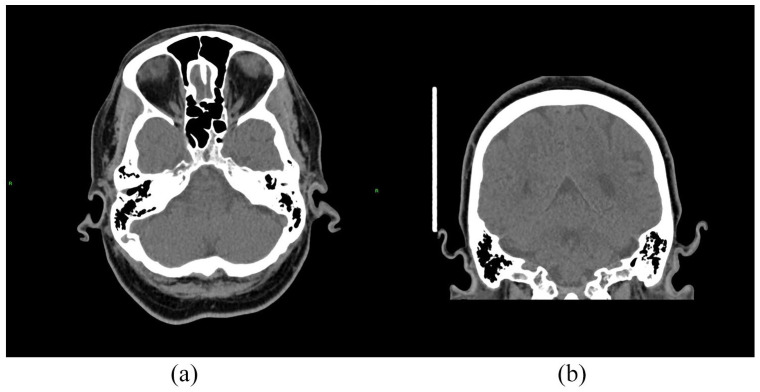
Thickening of the left pinna on head CT: (a) axial and (b) coronal.
